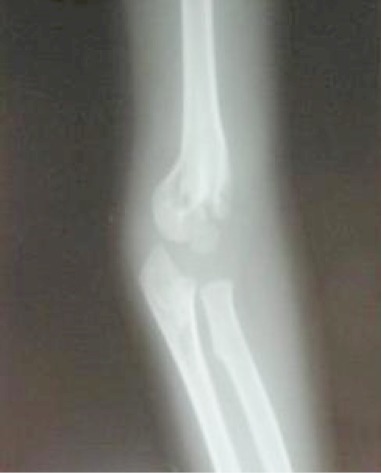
Radiographie de face et de profil du coude droit montrant une fracture supra condylienne stade II
